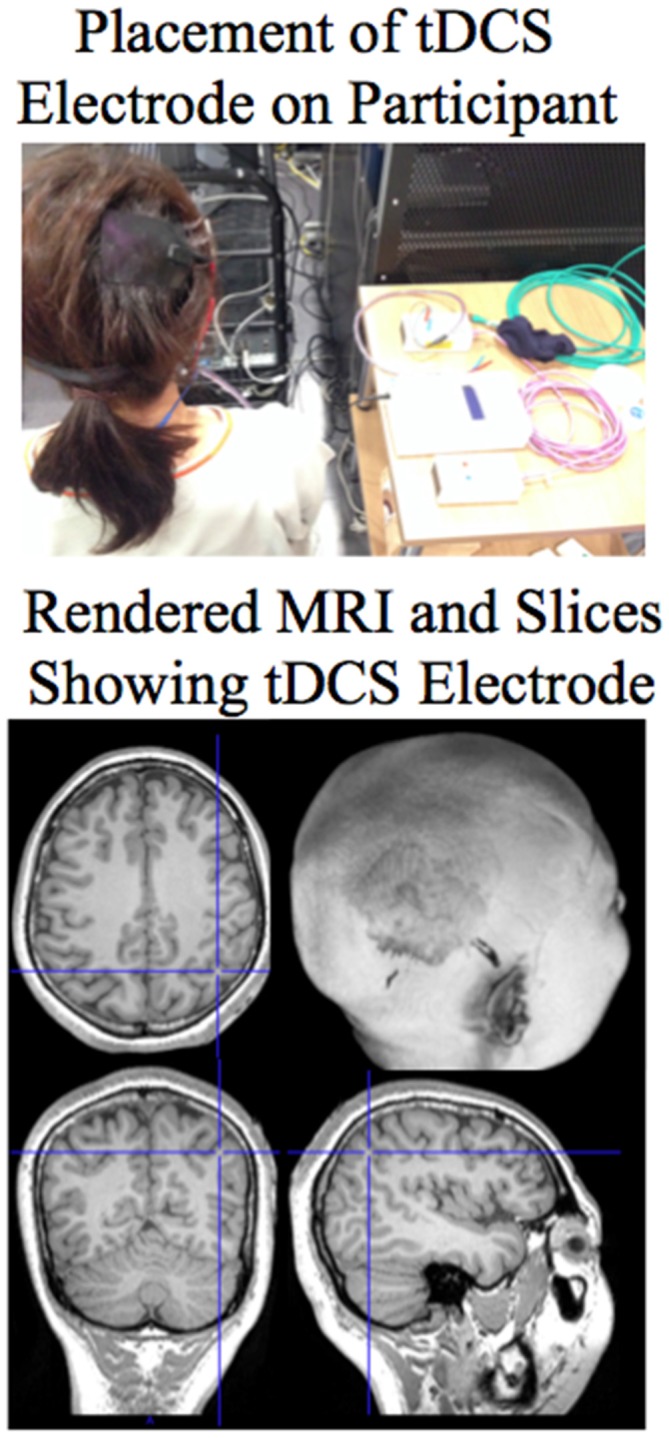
Top: Picture showing the placement of the anodal tDCS electrode on the right posterior parietal cortex of the participant. Bottom: The placement of the tDCS electrode can be seen in the rendered MRI of the participant. Sections are shown through the brain at the site of the electrode. For the MRI sections the right side of the image is the right side of the brain.
